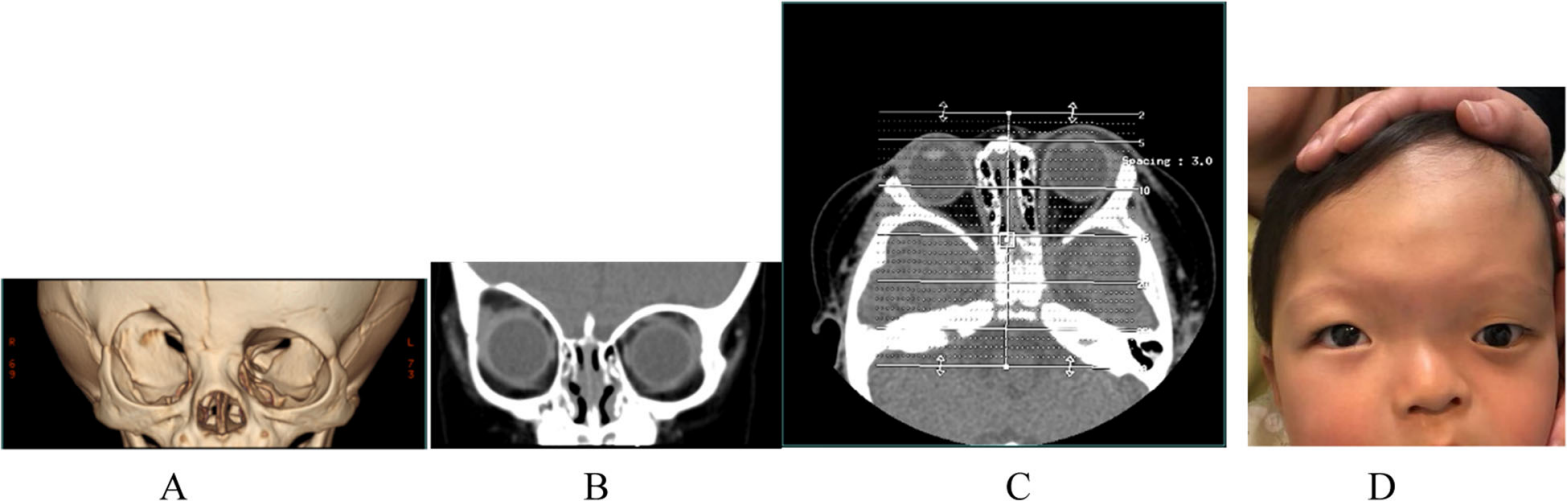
a The three-dimensional reconstruction of the computed tomography (CT) scan of the child’s skull with right UCS; b: Coronal view; c: Axial view; d: Right UCS with LMED
